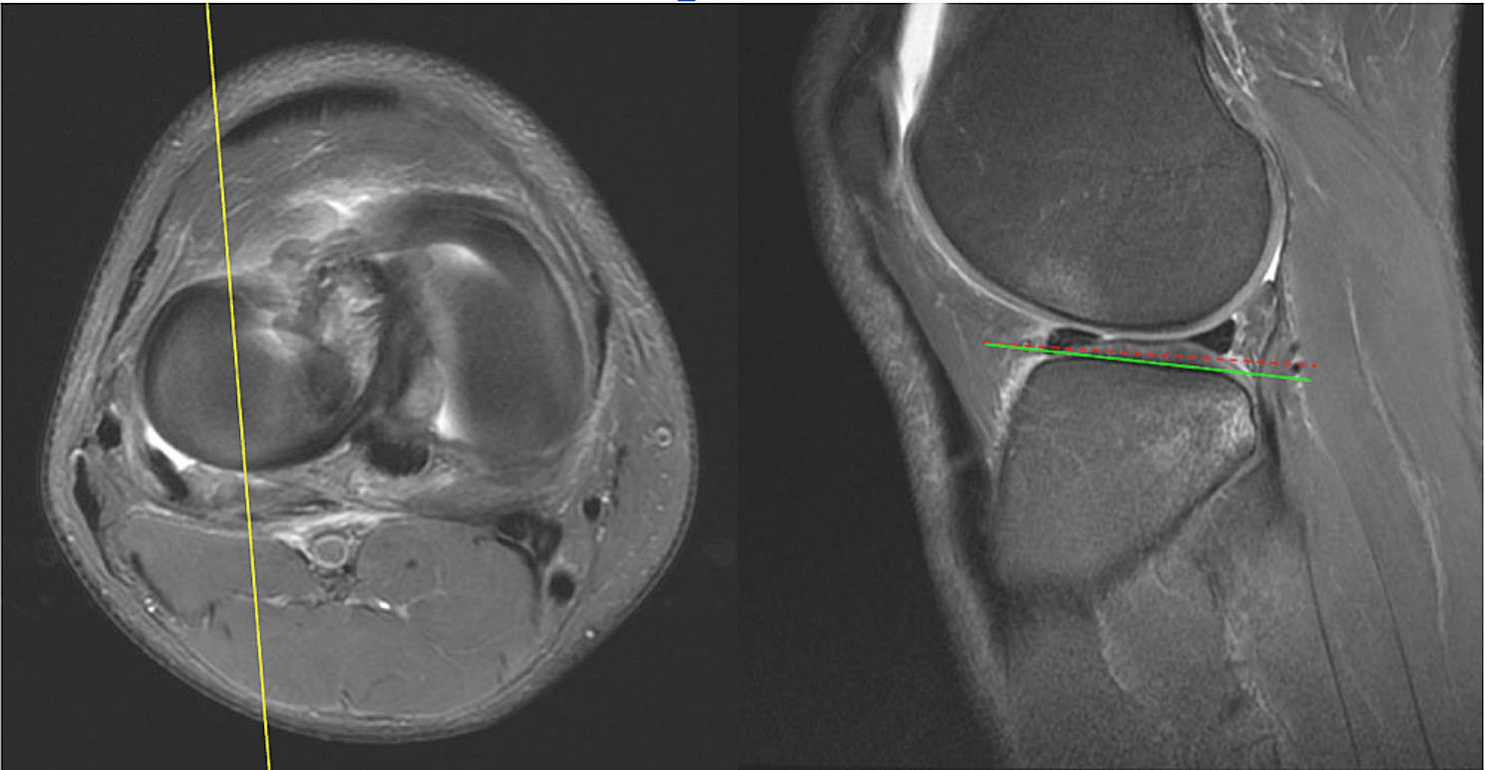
Measuring the lateral tibial slope: The reference line is adjusted to the center of the lateral tibial plateau. Connect the peak points of the anterior and posterior aspects of the plateau on the Sagittal MRI (green line). The angle between the green line and the dotted red line is defined as the lateral tibial slope
